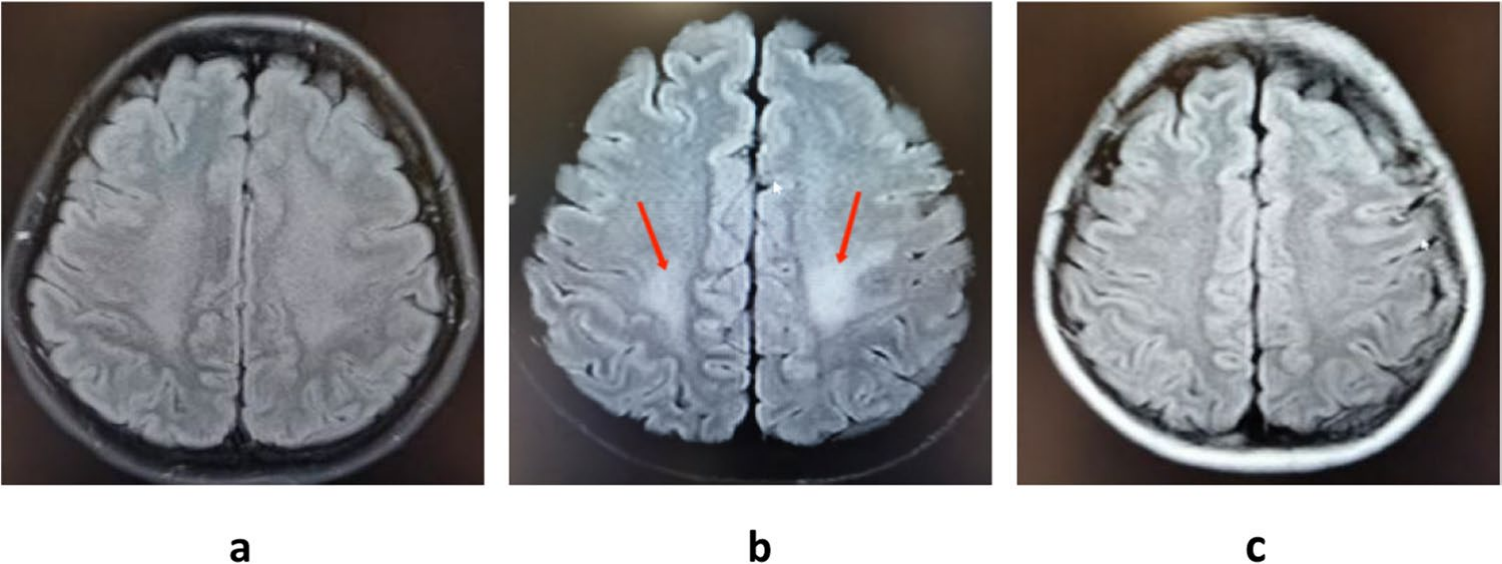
aNormal MRI on September 3, 2021;bdemyelinating lesions in the centrum ovale majus and bilateral ventricle on September 30, 2021;clesions dissipation after IVIG and prednisone on November 1, 2021
